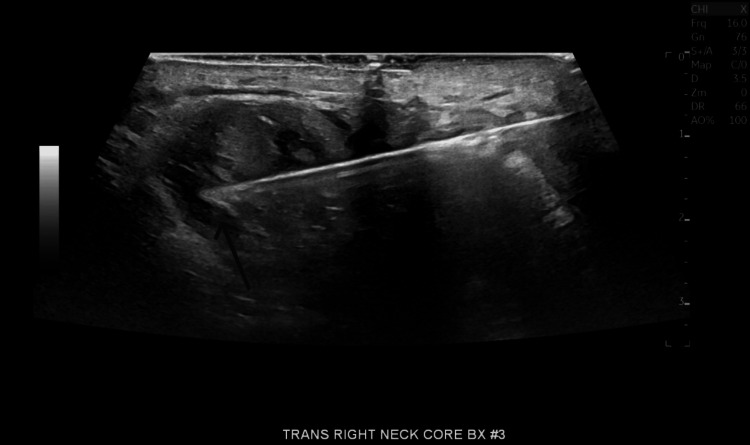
Ultrasound-guided biopsy of neck lump
